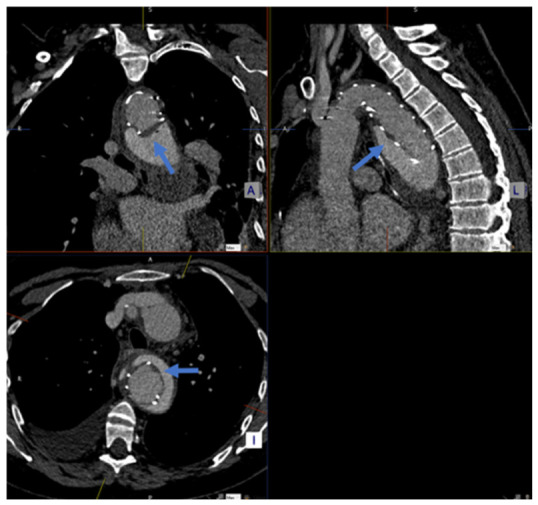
Computed tomography angiography (CTA) demonstrating intraluminal thrombus (ILT) within a frozen elephant trunk graft. This representative axial CTA image shows the characteristic appearance of intraluminal thrombus formation within the stent graft component of a frozen elephant trunk prosthesis (ILT shown as hypodense/dark area indicated by blue arrow).
